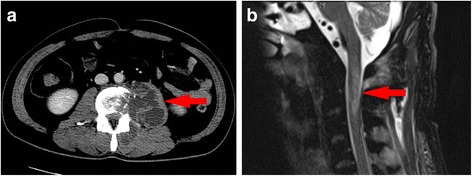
A psoas abscess and its remote involvement. a Ring enhancement in the left psoas muscle on an enhanced CT image obtained on the day of admission. b The cervical MRI findings revealed altered signal intensity involving C2-3, with cord expansion on T2-weighted sagittal sections, suggestive of myelitis
